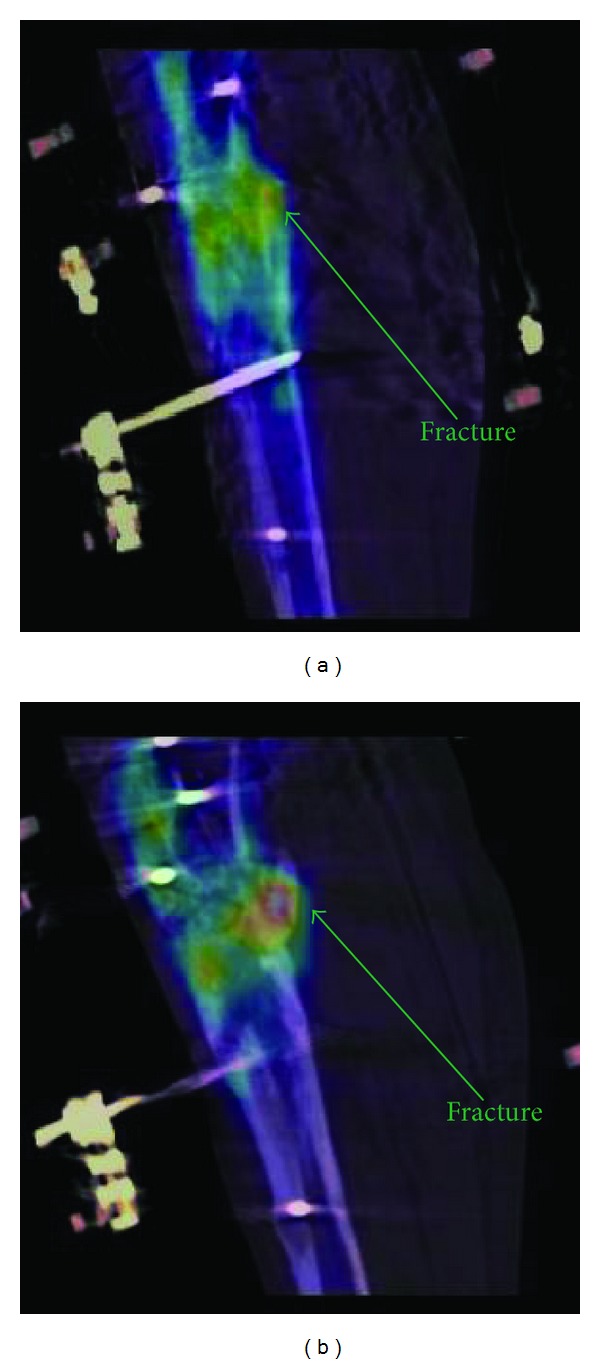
A sagittal fused PET/CT section of the crural remodeling area for Patient 1 at 40 days (a) and at 84 days (b) after the initial operation to attach the TSF. The crural remodeling region on the PET is better aligned to the CT in (a) than in (b).
